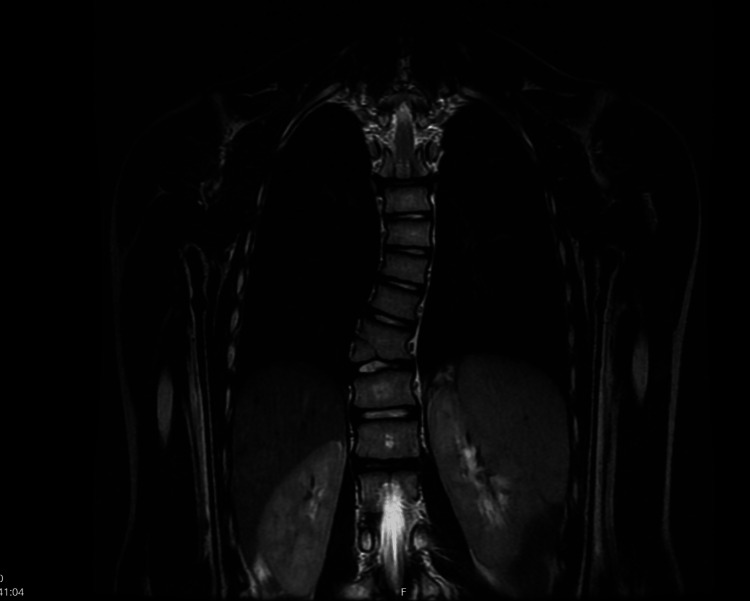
Magnetic resonance image showing congenital scoliosis.
